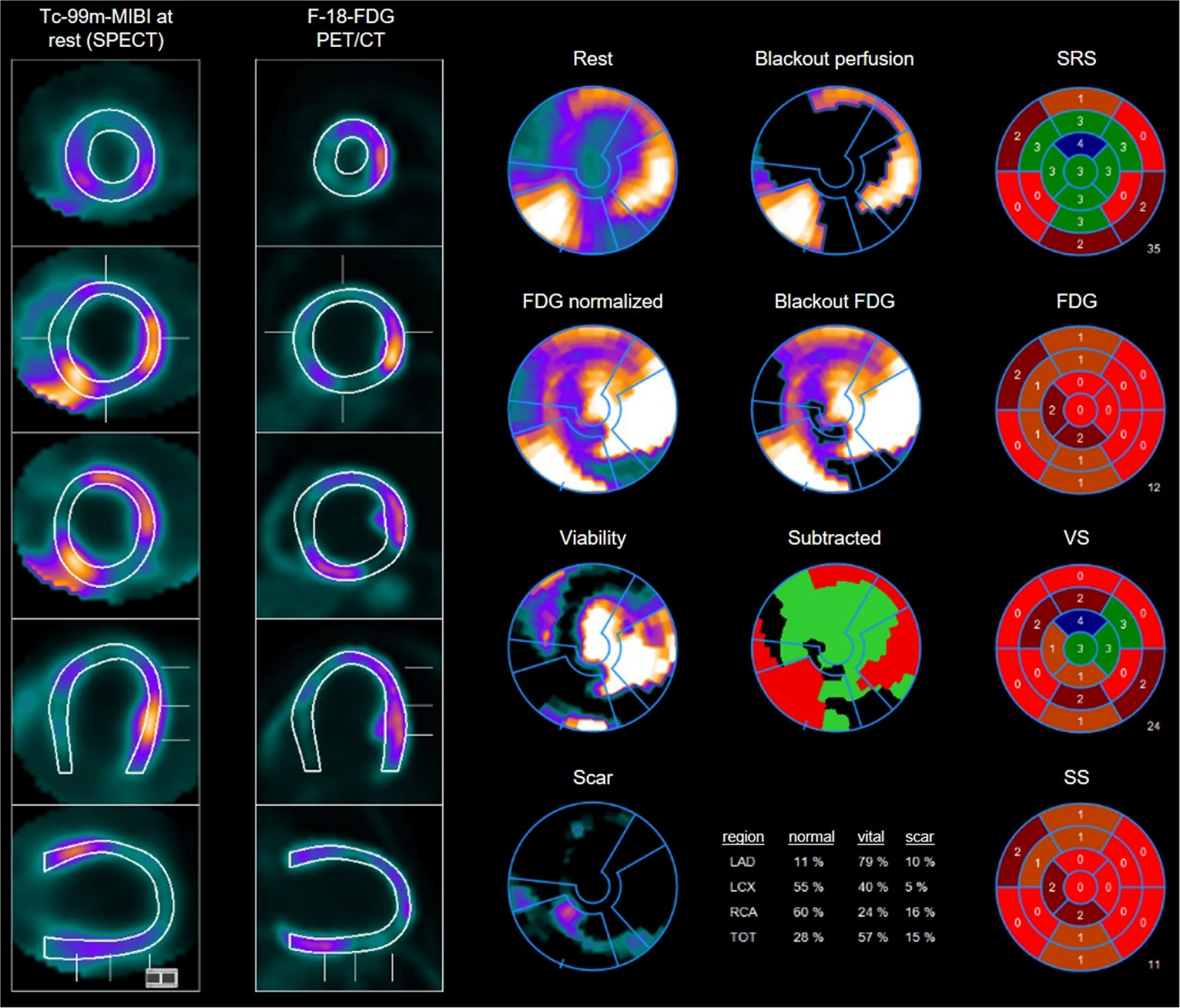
Example of multiple perfusion defect with hibernating myocardium as well as areas of scars: 45 years old male with perfusion defects in the anteroseptal wall as well as on the anterolateral and posterolateral wall in the Tc-99m-MIBI rest scan. FDG uptake in the viability F-18-FDG-PET/CT scan shows some uptake in the areas with perfusion defects resulting in the diagnosis of hibernating myocardium but also some areas with no FDG uptake especially septal and posterior resulting in the diagnosis of scars. Overview and polar maps with SRS, summed rest score; VS, vitality score; SS, scar score.
